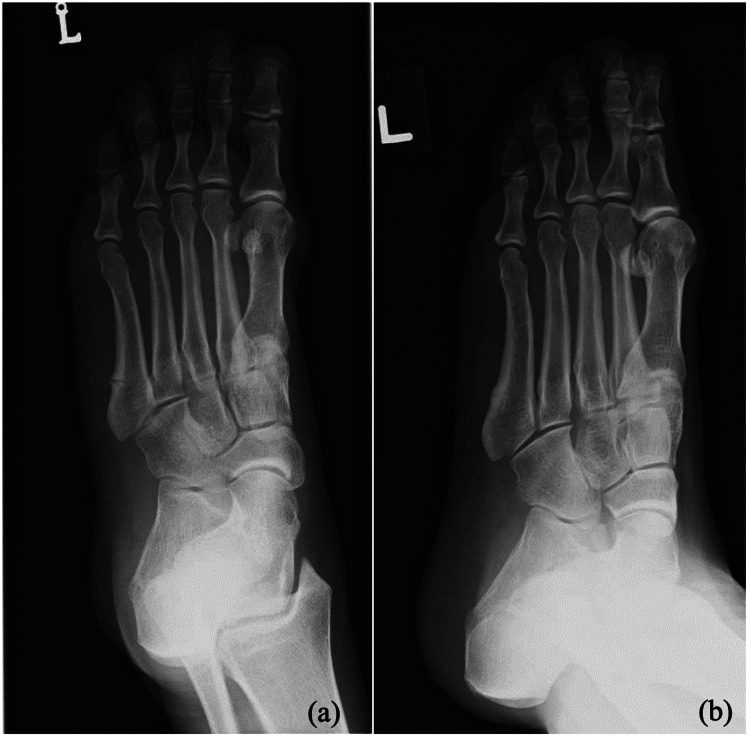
Radiographs of the left foot showing the Jones fracture in February 2019. (a) At initial presentation and (b) after six months
